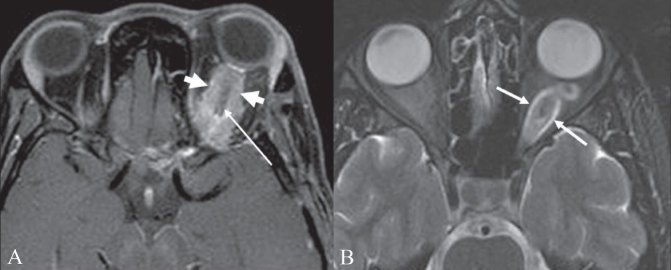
Optic nerve tram-track sign. Postcontrast T1W axial MRI image of the orbits (A) shows an enhancing mass (thick small arrows) around the left optic nerve. The optic nerve (thin long arrow) itself is not enlarged and is nonenhancing. This is suggestive of a mass, such as a meningioma, arising from the optic nerve sheath. Axial T2W MRI image of the orbits in a different patient (B) shows fusiform enlargement of the left optic nerve itself (arrows), in a case of optic nerve glioma
